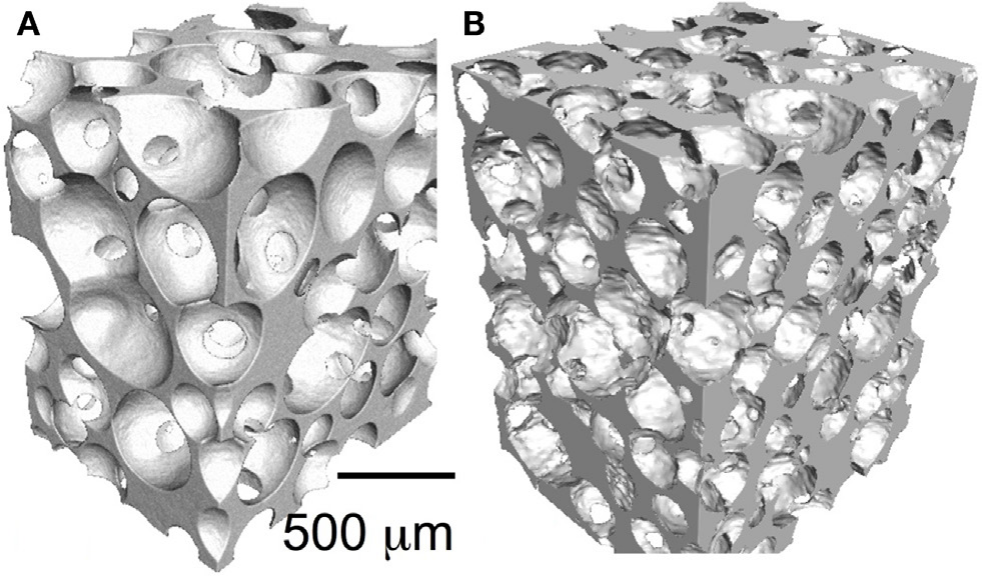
X-ray microtomography images of bioactive glass scaffolds (A) sol–gel foam and (B) melt-derived gel-cast foam. Modified with permission from Jones (2013).
